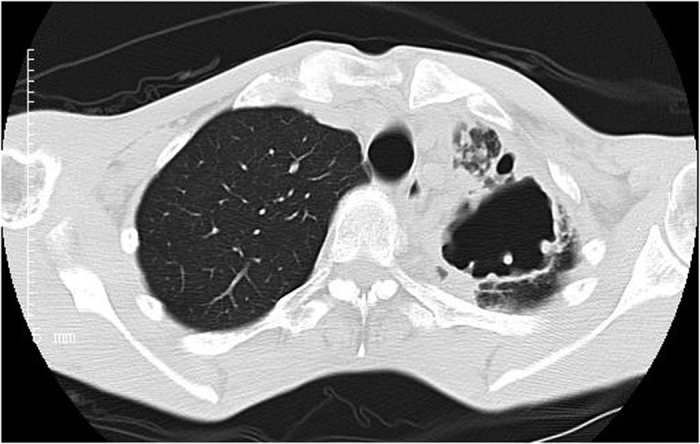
M. szulgai* lung disease in a 38-year-old man. Chest CT scan shows a large cavity and centrilobular nodules in left upper lobe and pleural thickness.
